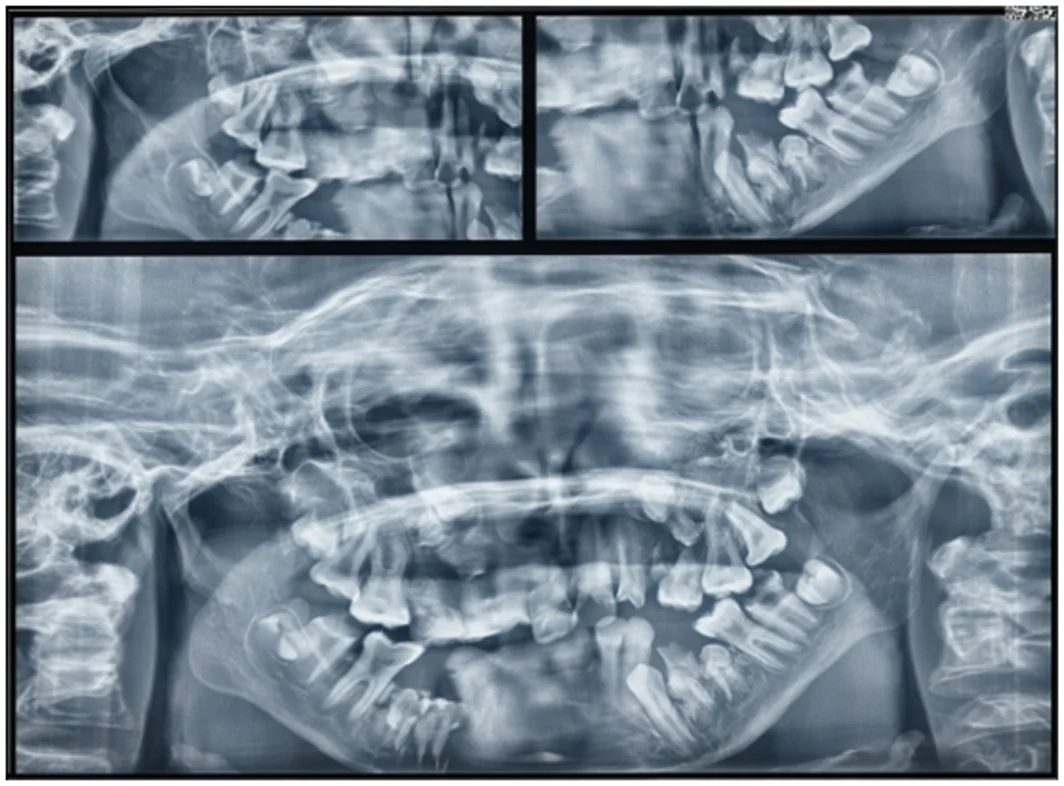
Initial panoramic radiograph (OPG) obtained in 2021 (1400 SH).
